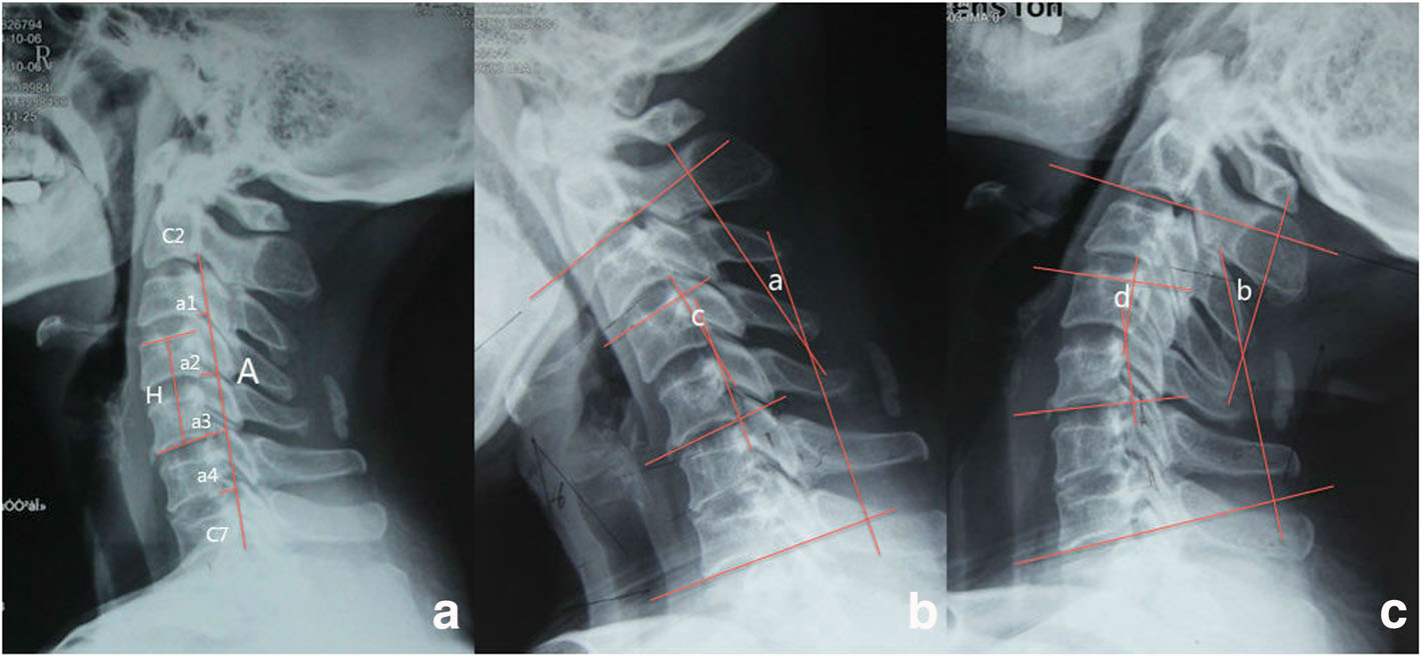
Lateral radiograph showing evaluation of the cervical curvature index (CCI = (a1 + a2 + a3 + a4)/A), intervertebral height (H), the ROM of cervical spine (a + b), and the ROM of FSU (c + d)
